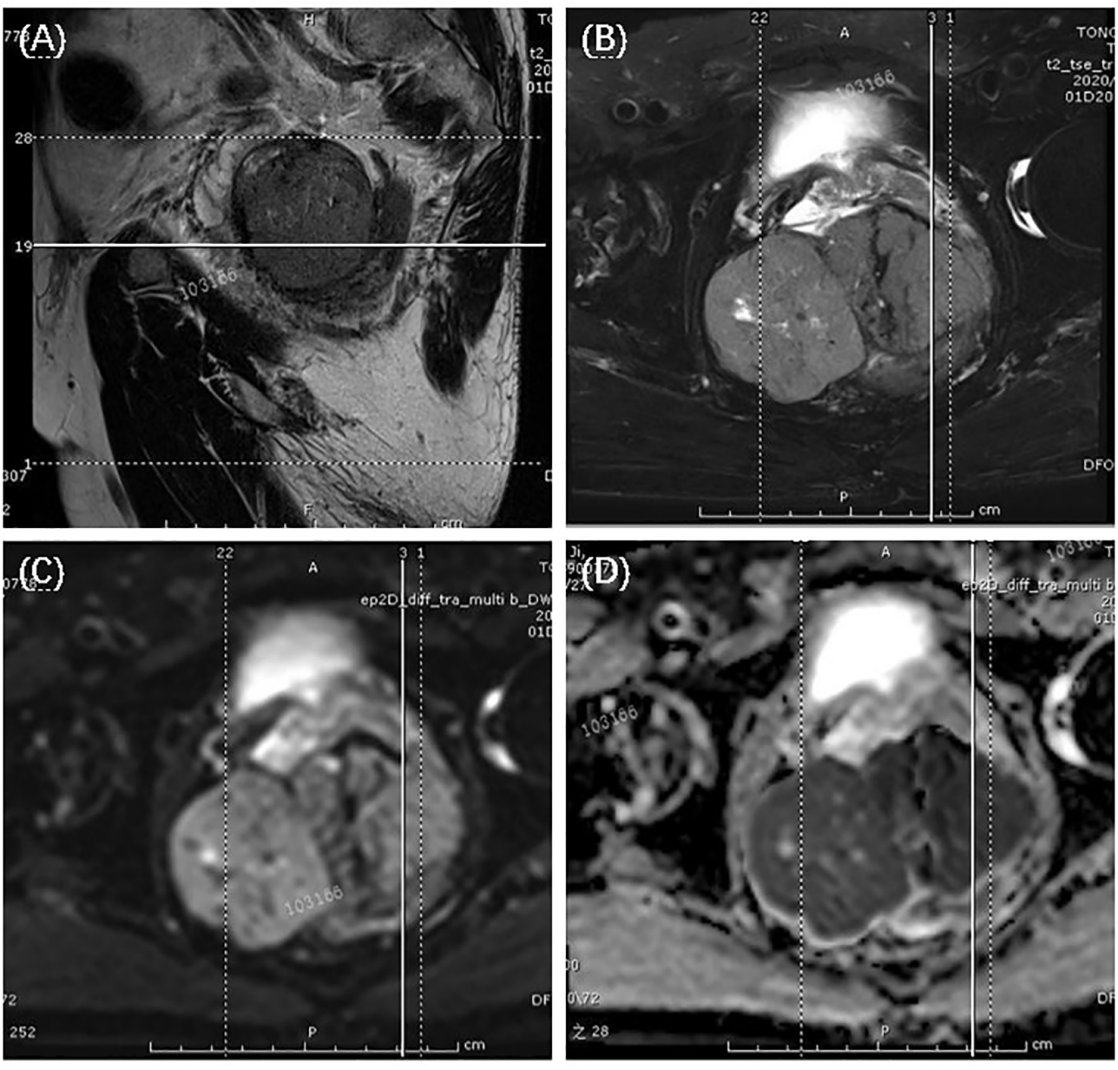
T2-weighted magnetic resonance imaging showed a large uneven tumor mass at the prostate with an unclear border with the seminal vesicles and anterior rectum, tumor appeared hyper-intensive. The arrows suggested the tumor. (A) sagittal view; (B) transection view; (C) diffusion-weighted imaging (DWI); (D) apparent diffusion coefficient (ADC) maps.
